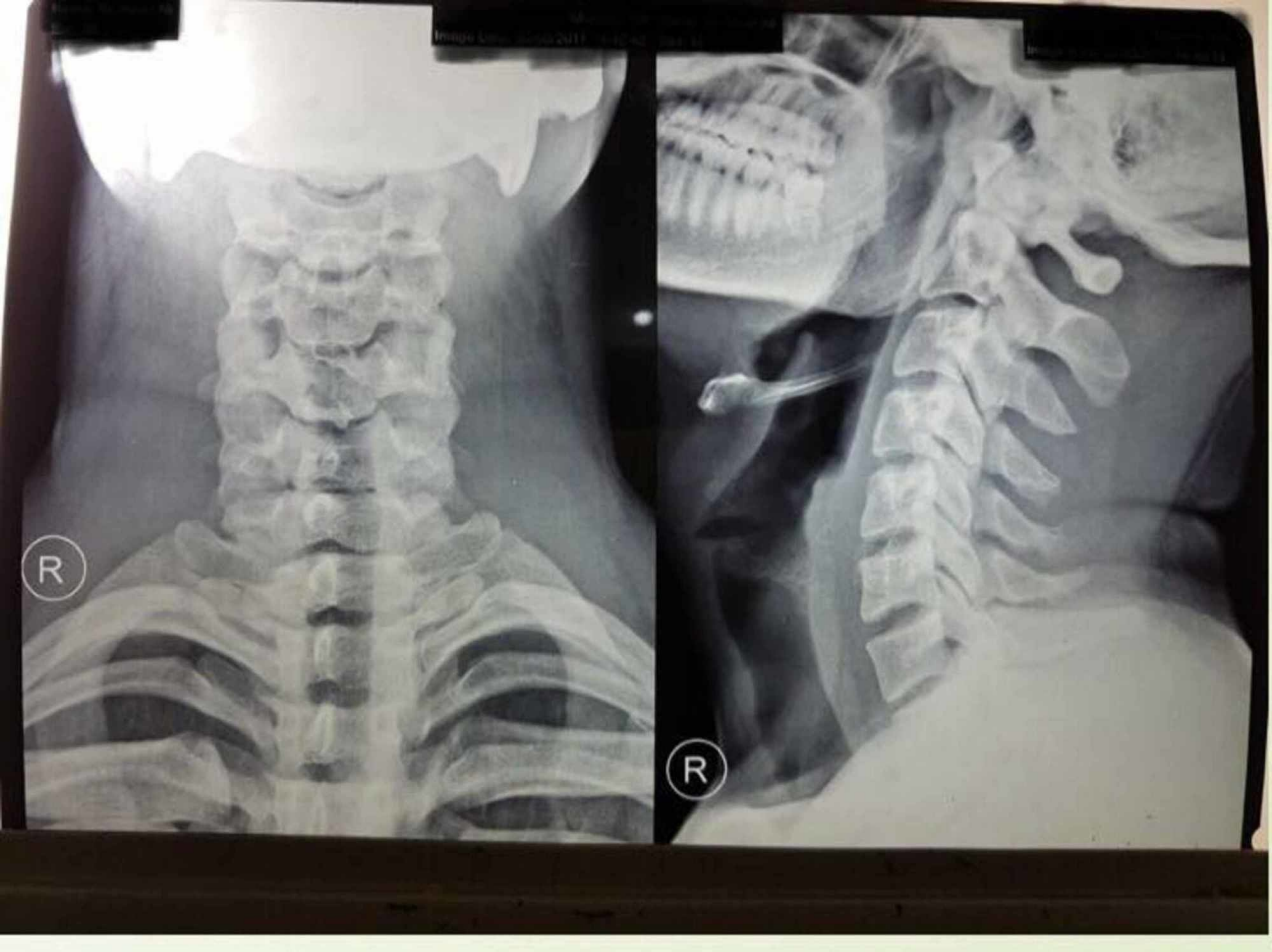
X-ray cervical spine of the patient
